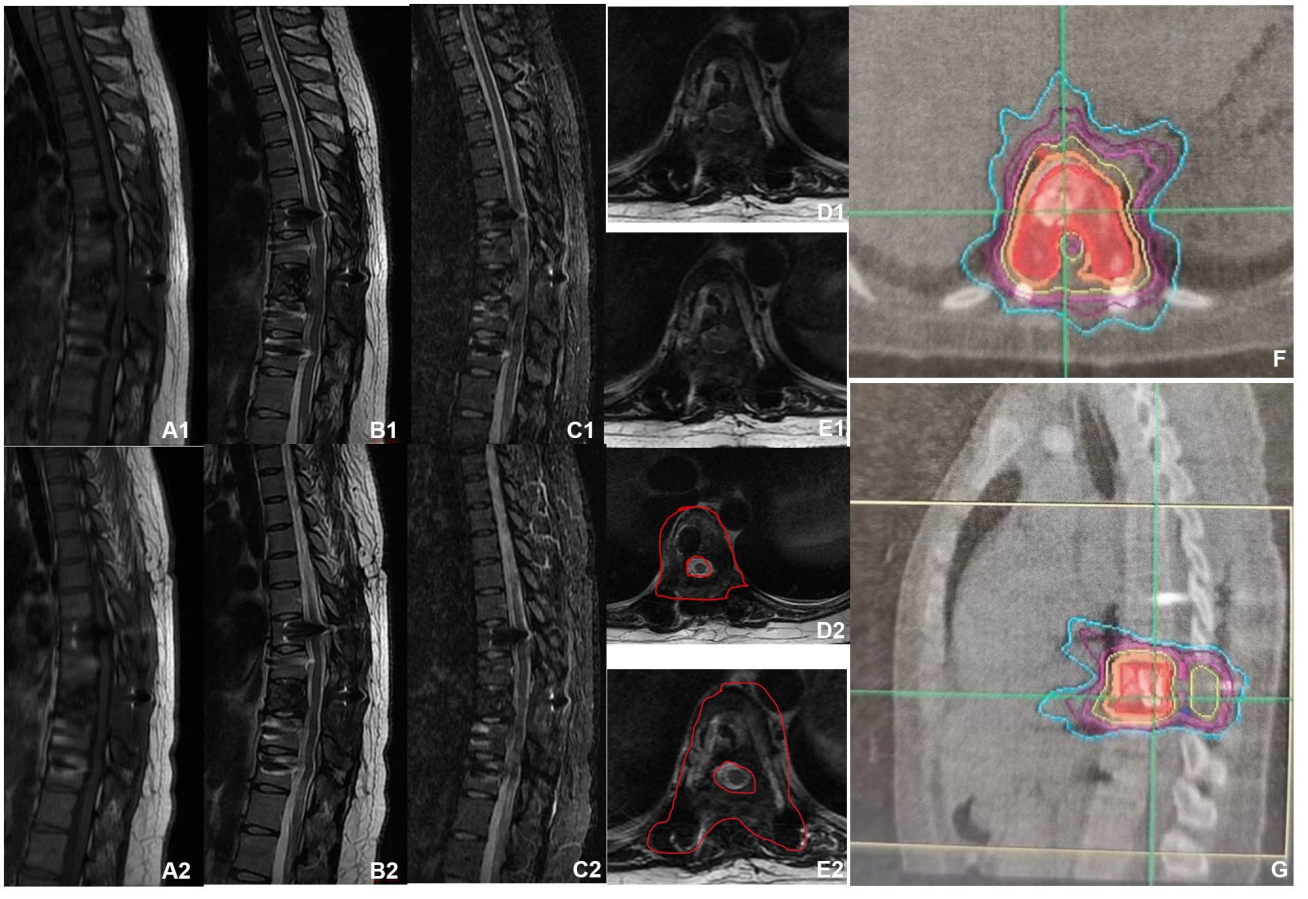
MRI before and after denosumab administration. (A1–E1) MRI before denosumab administration showed uneven bone signals in the T9 vertebrae, with patchy abnormal signals at the posterior margin of the vertebral body. Burst into the spinal canal, and spinal cord compression can be seen. (A2–E2) MRI of the thoracic spine after three doses of denosumab administration (2021-02). The space in the spinal canal is significantly smaller than that at the front, and the boundary with the spinal cord is clear. (F,G) Preoperative planning for cyber knife radiation therapy.
